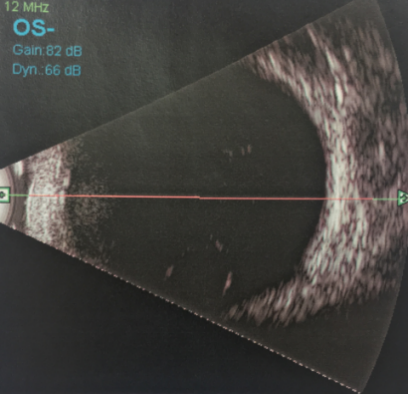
B-scan ultrasound of the left eye with vitreous opacity
